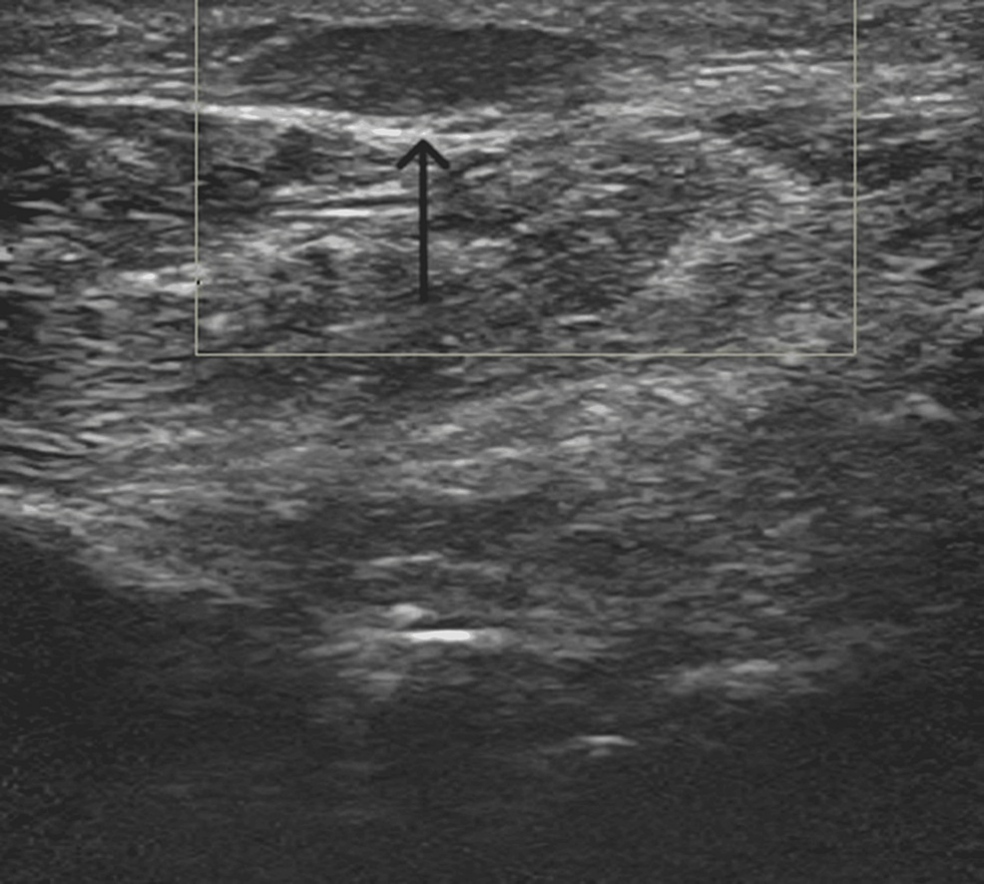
An ultrasound of the arch of the left foot was performed and found a hypoechoic and homogeneous nodule within the plantar fascia. Color and power Doppler imaging revealed no internal blood flow.
